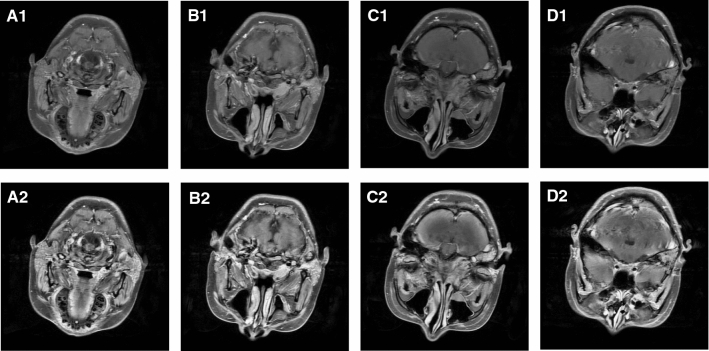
MRI examples before and after preprocessing. The images in the first row are original ones, while the images in the second row are processed using CLAHE
